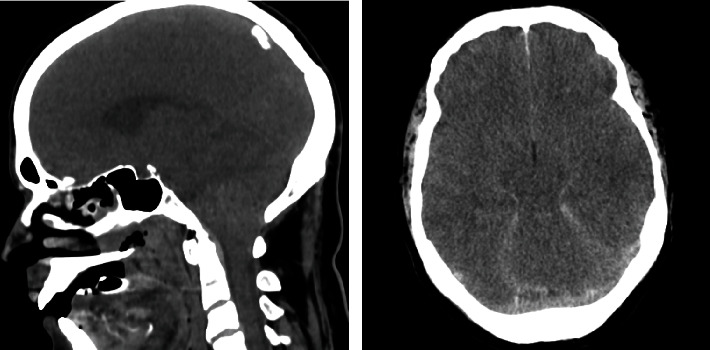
Sagittal and axial views of cranial computed tomography imaging showing diffuse cerebral edema, with loss of grey–white matter differentiation, obliteration of cisterns and sulcal spaces, and cerebellar tonsil herniation.
